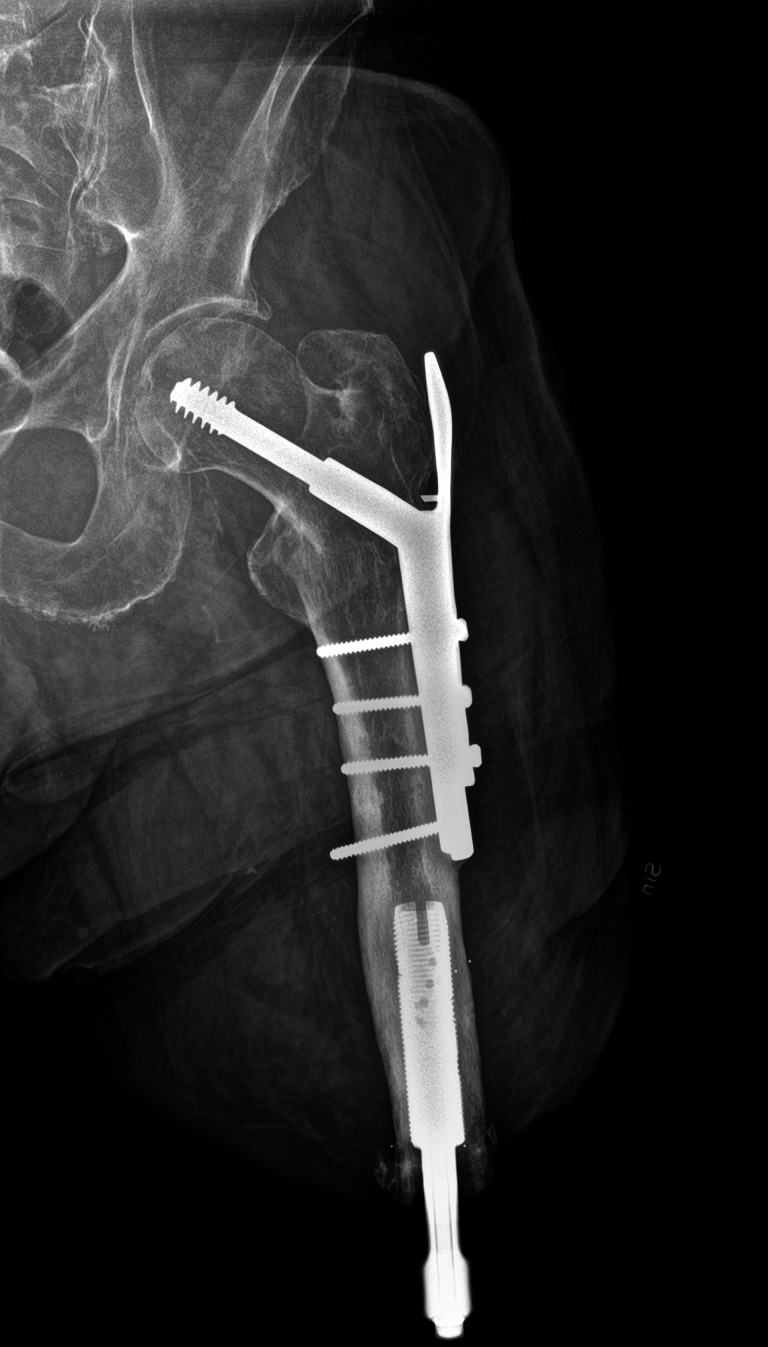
An example of a X-ray image of a pertrochanteric femur fracture fixated with osteosynthesis on the ipsilateral side as the BAP. BAP, bone-anchored prosthesis.
